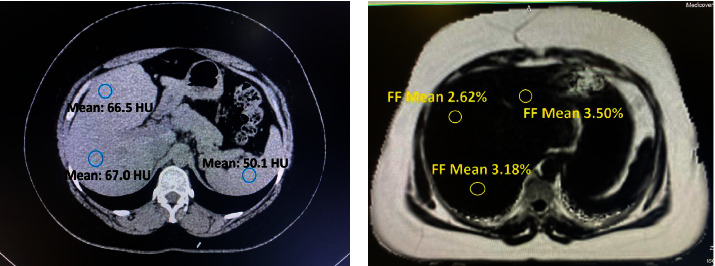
(a) LAI +16 on plain CT scan. (b) MR fat fraction 2-3% in both lobes (LAI = liver attenuation index).
